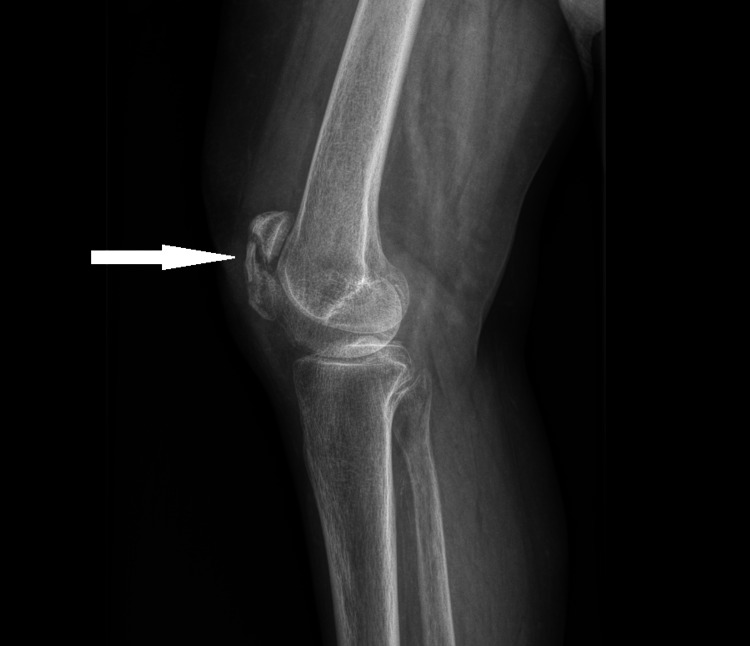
Sagittal view X-ray of the patient's right knee demonstrating a comminuted fracture of the patella, with a 4 mm distraction of the superior pole, overlying soft tissue swelling, and an associated joint effusion.
